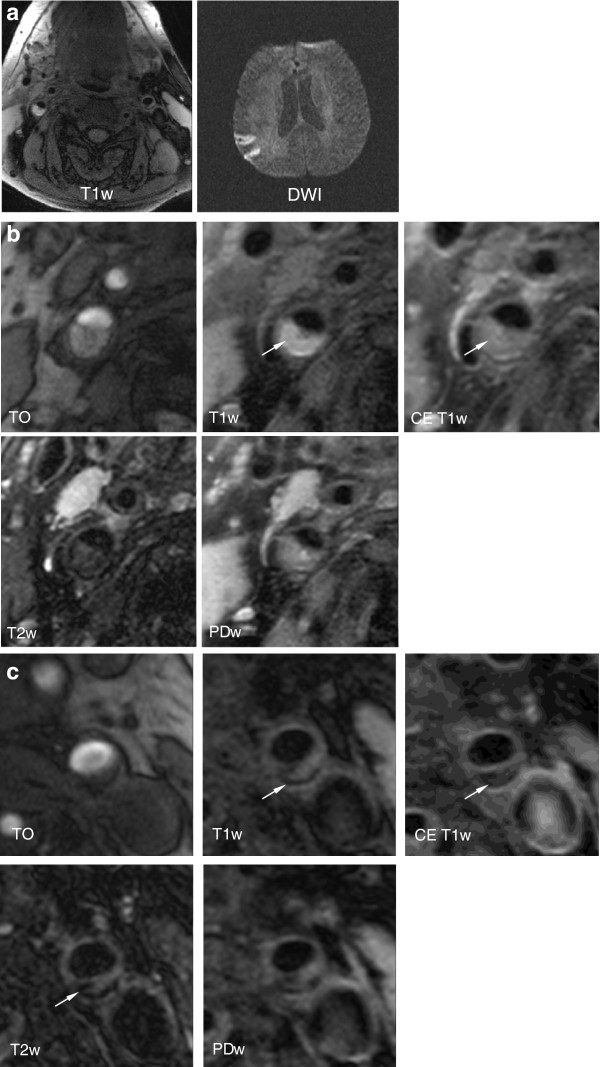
Imaging example. Figure 1 shows various CMR-images of a 66 years old patient who suffered an infarction 4 days before the CMR scan. a. T1w and DWI MR-images of the patient. Axial T1w images with fat suppression demonstrate atherosclerotic lesions in both internal carotid arteries (ICA). The lesion in the right ICA, ipsilateral to the symptoms, is hyperintense, consistent with intraplaque hemorrhage. The lesion in the left ICA is hypo- to isointense, consistent with a fibrous and calcified lesion. Axial DWI images show a diffusion restriction in the posterior part of the right middle cerebral artery, consistent with an acute brain infarction. b. Axial multi-sequence CMR images of the same patient demonstrating the complicated AHA lesion type VI plaque ipsilateral to the stroke in the right ICA. The arrow points to a region which is hyperintense on T1w and TOF images and hypointense on PDw and T2w images, consistent with type I hemorrhage into a large necrotic core. The dark band on TOF images is disrupted, the hyperintense area on TOF is located juxtaluminally and the fibrous cap cannot be visualized in the contrast enhanced T1w images, indicating rupture of the fibrous cap (ICA = Internal carotid artery, TOF = Time-of-Flight). c. Axial multi-sequence CMR images of the same patient demonstrating the uncomplicated AHA lesion type VII plaque contralateral to the stroke in the left ICA. The arrow points to a region which is hypointense on all images, consistent with a calcification, surrounding an area which is isointense to fatty tissue in T1w, T2w and PDw images and shows no contrast enhancement, consistent with a necrotic lipid core. The contrast enhancing fibrous cap separating the plaque from the lumen appears intact. (ICA = Internal carotid artery, TOF = Time-of-Flight).
